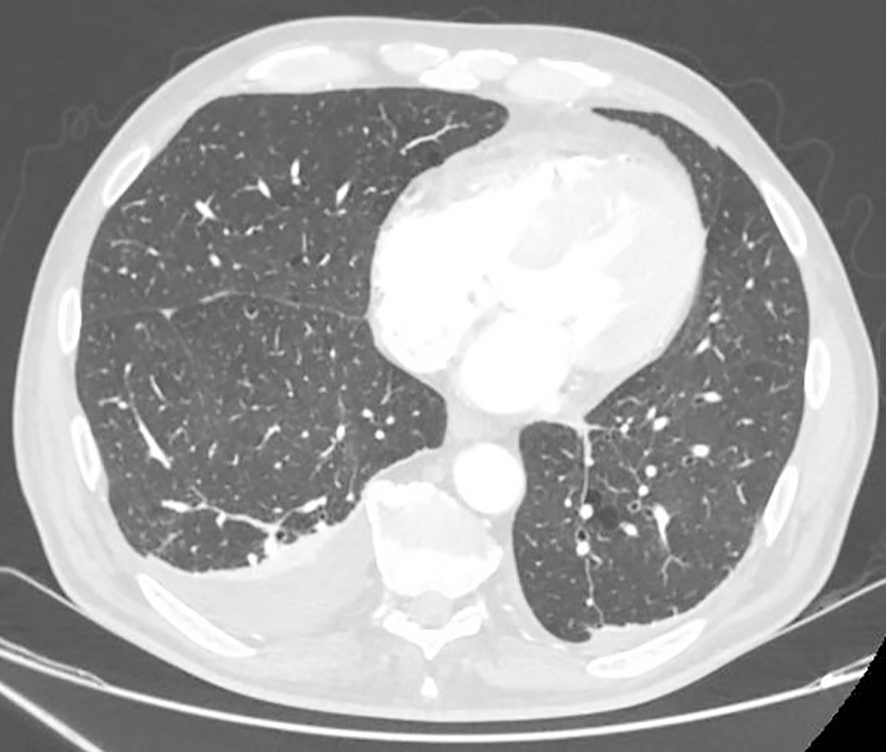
Computed tomography imaging revealed bilateral pleural effusions, with greater accumulation on the right lobe.
